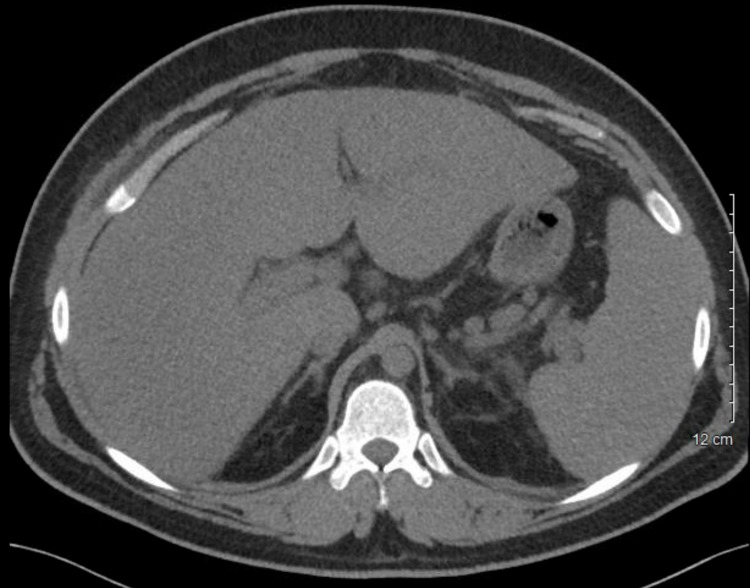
Non-contrast abdominal computed tomography obtained on admission showing hepatomegaly and splenomegaly.
